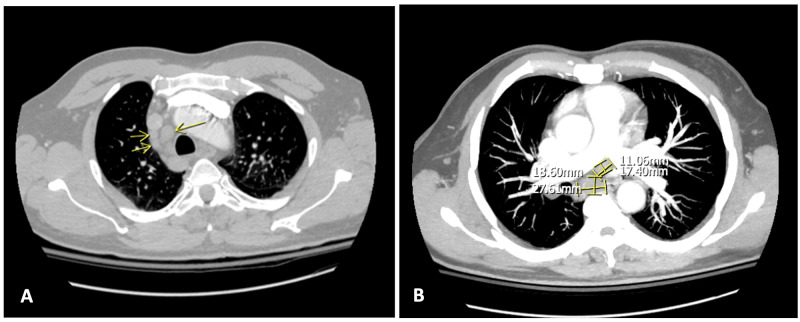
Axial images (A and B) from a CTA demonstrate mediastinal adenopathy with the largest node measuring 27.6x18.6 mm in the subcarinal region. CTA: computer tomography angiography
